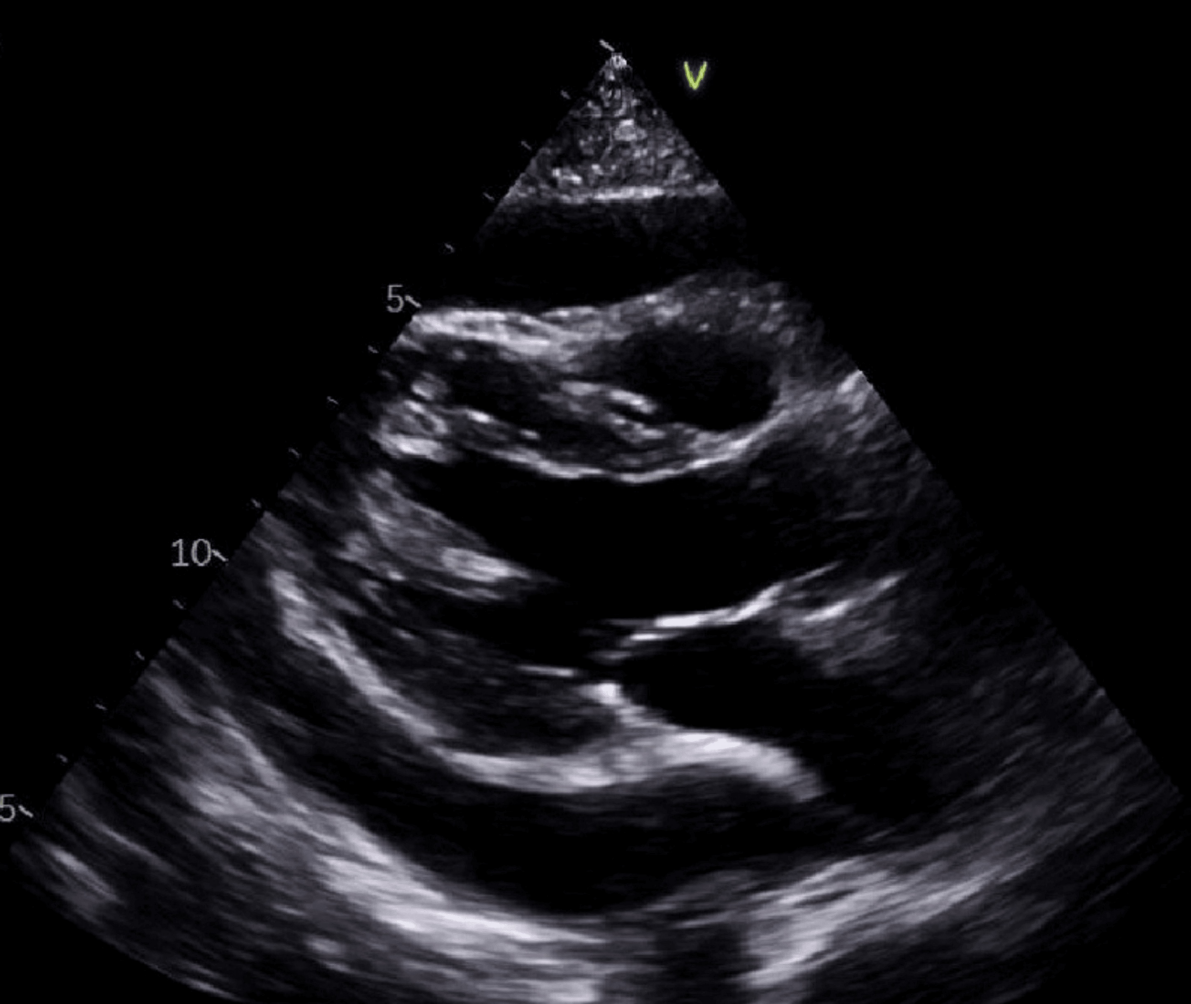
Parasternal long-axis view obtained prior to pericardiocentesis, showing a large pericardial effusion with a maximum measured dimension of 23 mm along the posterior wall.
